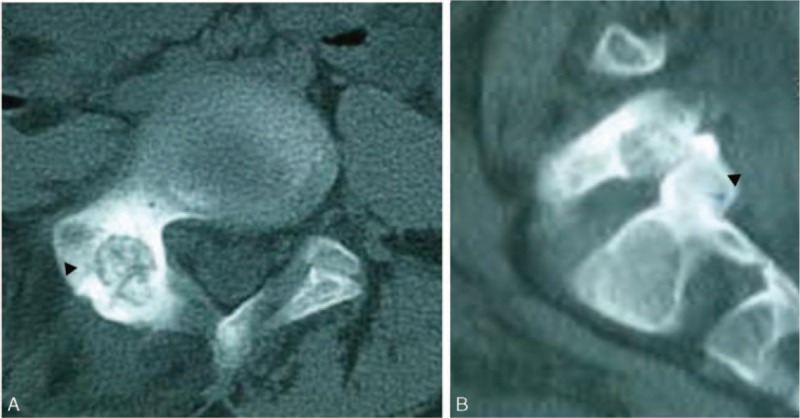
A, CT revealed a mass lesion at the right lamina of the fifth lumbar vertebra. Punctate calcification was observed within and around the lesion; reactive sclerosis change was observed around the lesions. B, Sagittal reconstruction CT showed lesions involving the right side of the fifth lumbar facet. CT = computed tomography.
